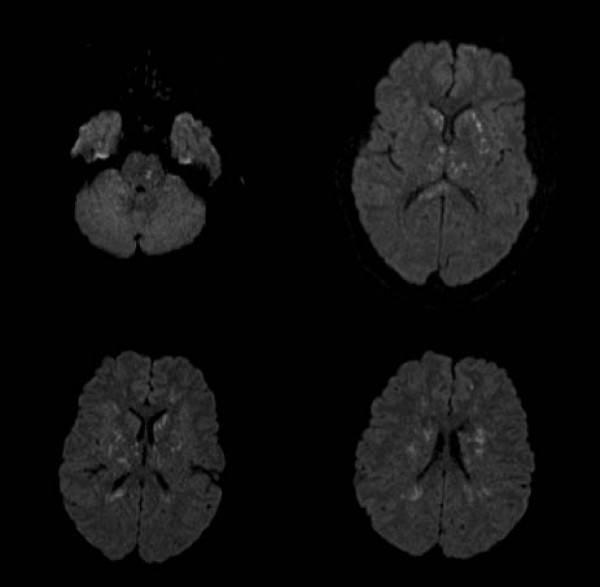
MRI shows punctate areas of restricted diffusion within the basal ganglia, thalami, splenium of the corpus callosum, deep white matter, frontoparietal cortex and pons.
